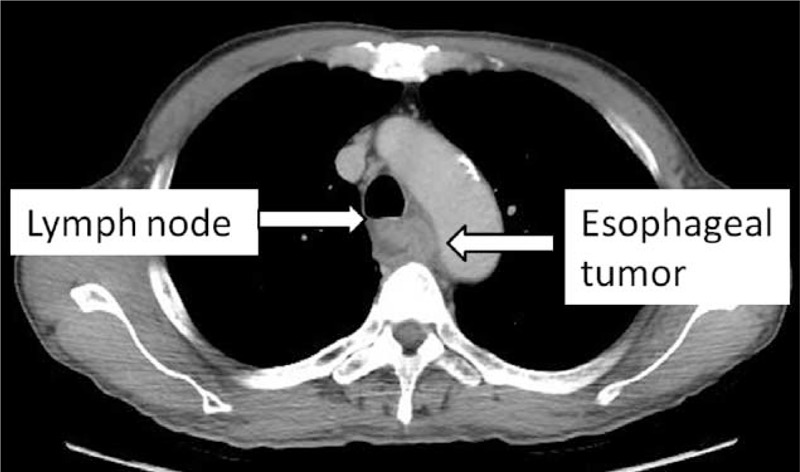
Chest computed tomography (CT) shows an esophageal tumor with obstruction at the middle third portion of the esophagus invading the adventitia. Also note the enlarged lymph node between the esophagus and the distal trachea.
